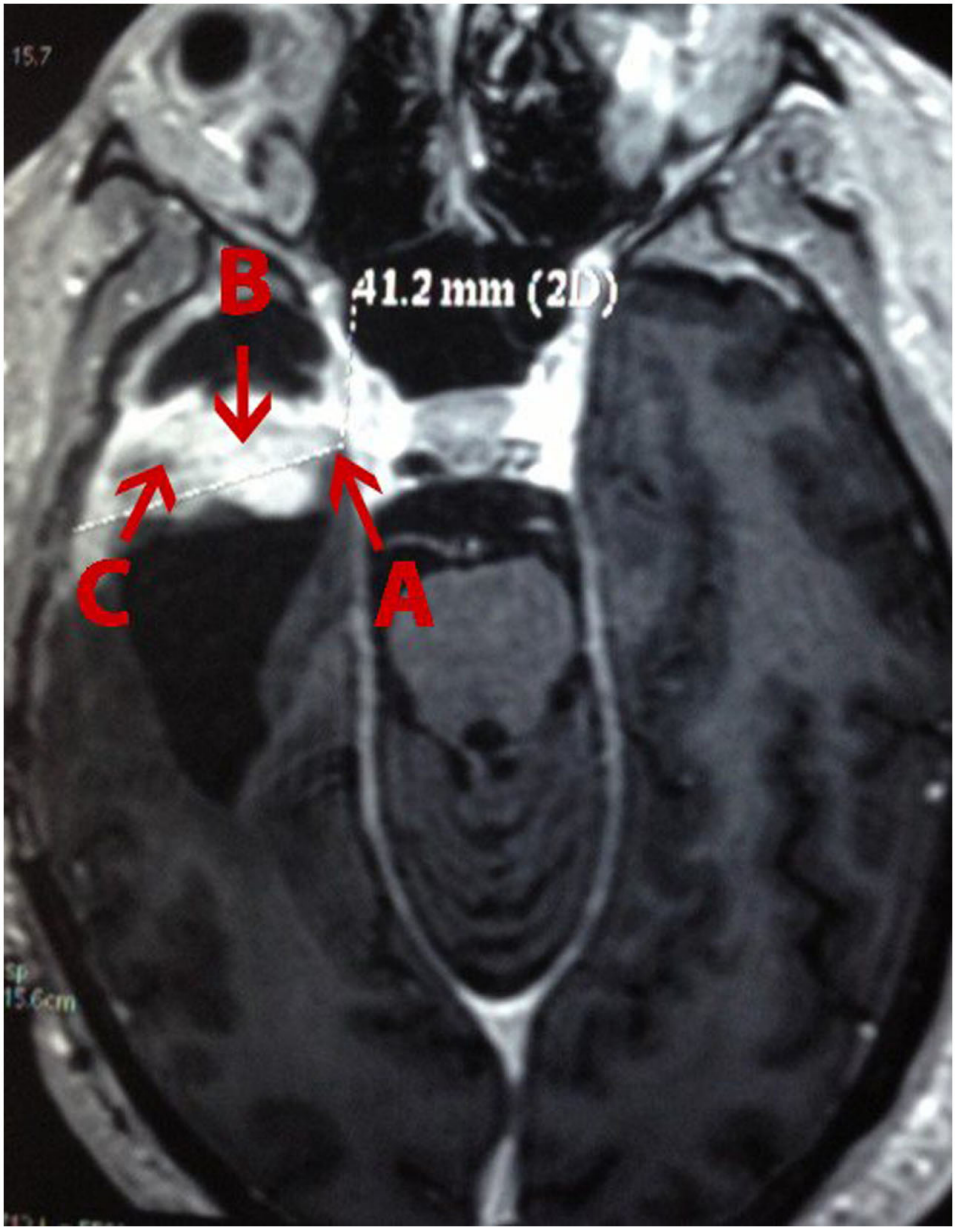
Magnetic resonance imaging (MRI) of a patient with recurrent glioblastoma multiforme (GBM) in the right temporal lobe. (A), external; (B), profound; and (C), intermediary (as in according to the tumor’s center) area of the GBM.
